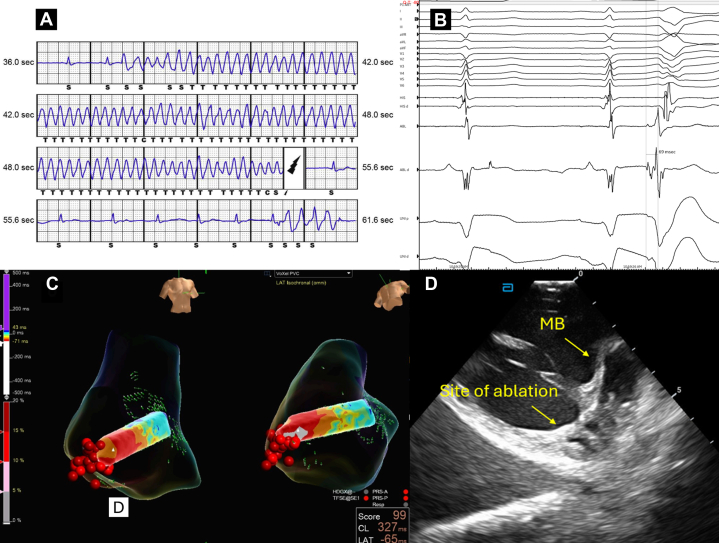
Example Case Example case of a 45-year-old gentleman with a history of an out-of-hospital cardiac arrest with documented VF who had recurrent appropriate ICD shocks (A). He had nonobstructive coronary artery disease with a structurally normal heart with focal nonspecific delayed gadolinium enhancement noted at the right ventricular insertion site and a variant of uncertain significance in alpha-protein kinase 3. He underwent ablation for PVC-triggered VF with earliest pre-QRS local ventricular electrogram (69 ms) (B) in the lateral aspect of the moderator band (C). Radiofrequency ablation followed by cryoablation was used with intracardiac echocardiography depicting the site of ablation (D). He was continued on flecainide 100 mg orally twice daily for 3 months postablation without clinical recurrence. ICD = implantable cardioverter-defibrillator; VF = ventricular fibrillation; other abbreviations as in Figures 1 and 6.
